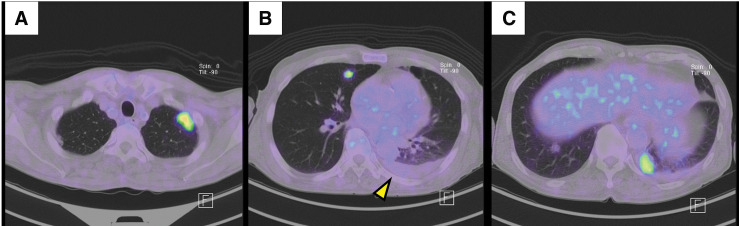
(A–C) Multiple pulmonary nodules uptook FDG, and left pleural effusion increased (yellow arrowhead). FDG, ^18^F-fluorodeoxyglucose
